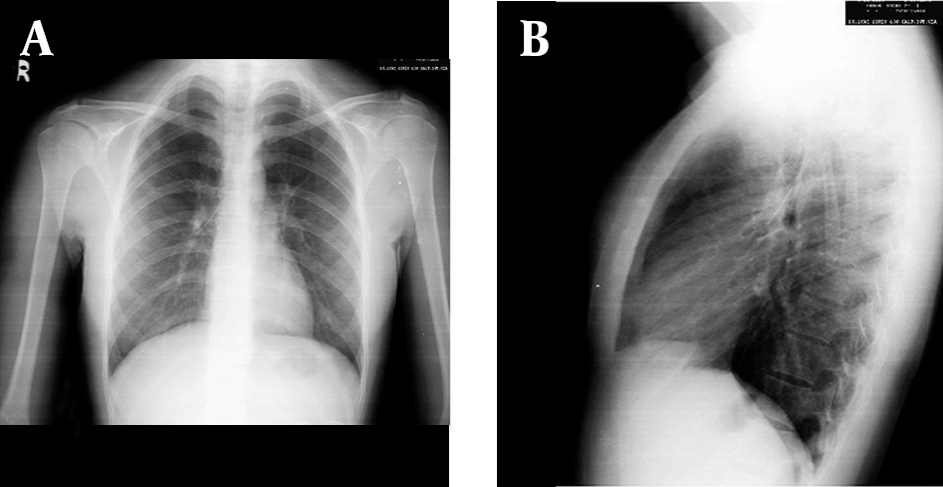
A. PA; B. right lateral Chest X-ray of the Patient Revealing a Radiopaque Foreign Material
